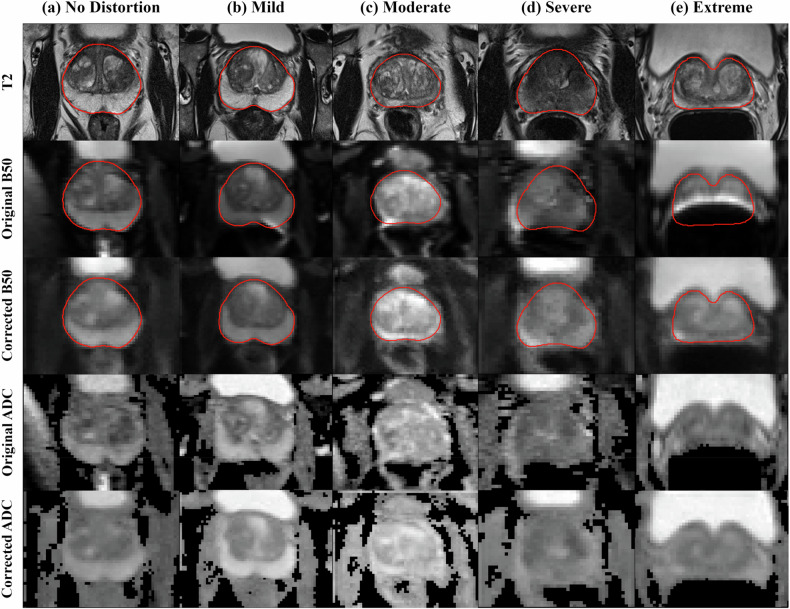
Representative exams across distortion severity levels. Each column shows a different subject from the test set, including (a) no distortion, (b) mild, (c) moderate, (d) severe, and (e) extreme distortion severity levels. Rows show the T2-weighted reference, distorted B50 diffusion-weighted images, DeDistortNet-corrected B50 diffusion-weighted images, and the corresponding apparent diffusion coefficient map. The red contour indicates the prostate mask derived from the T2-weighted image
